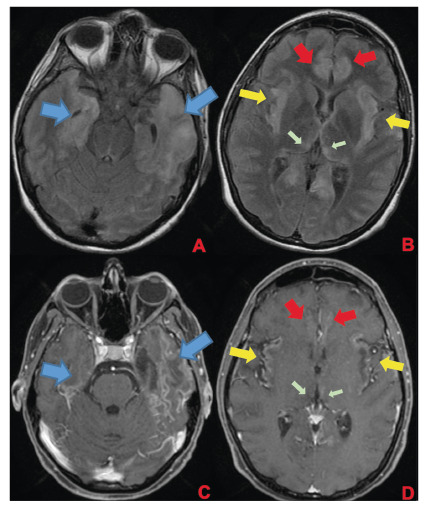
First brain MRI of the patient. In the superior row (A and B), axial FLAIR images; in the inferior row (C and D), axial T1 post-contrast images. Findings demonstrate encephalitis with involvement of limbic structures such as the temporal lobes (blue arrows), insula (yellow arrows), cingulate gyrus (red arrows), and medial portions of the thalami (green arrows). FLAIR images (A and B) show hyperintensities in cortical and subcortical brain parenchyma with a subtle mass effect, while post-gadolinium images demonstrate cortical enhancement in these regions and findings consistent with volume loss in the left mesial temporal lobe and insular cortex, likely resulting from previous encephalitis.
